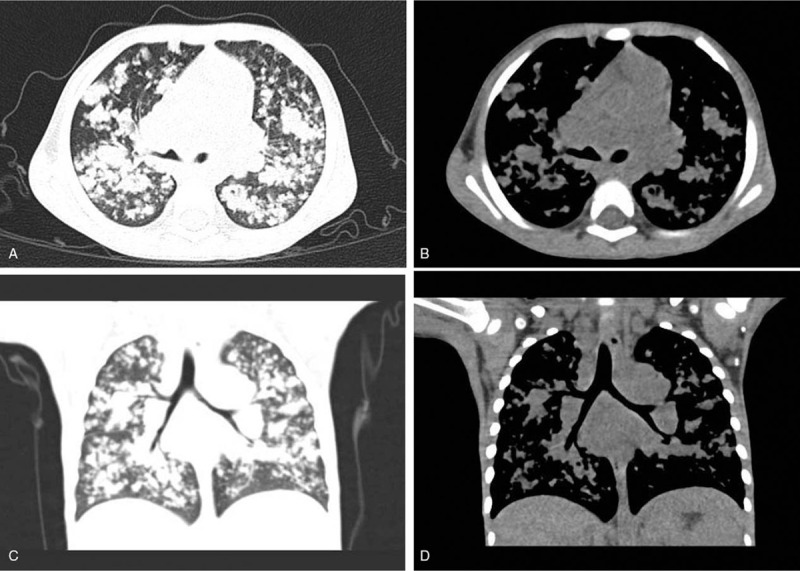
Male, 2 years old, recurrent fever for 1 month with aggravation and a cervical mass in 10 days. CT shows that both lungs have multiple nodular and patchy densities, which are mainly distributed along the bronchi in varied size. There is lymphadenectasis in both hila, mediastinum, bilateral supraclavicular regions and bilateral axilla. Among them, some enlarged lymph nodes are fused to form masses (A–D).
